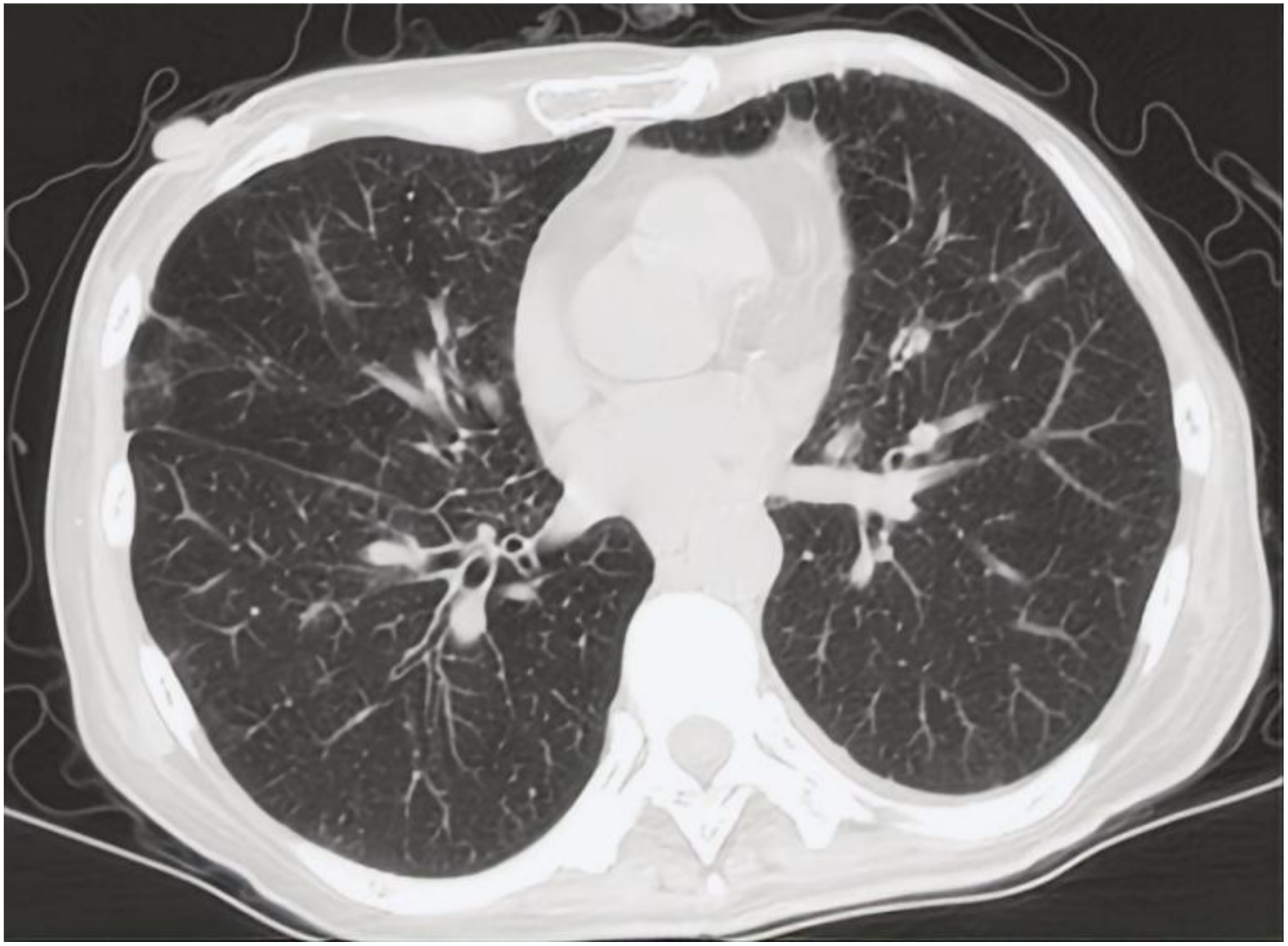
CT scan of chest showed multiple small nodules considered as metastatic carcinoma in both lungs.
